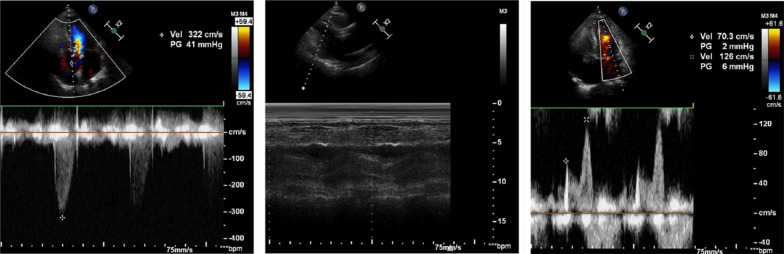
1 year follow-up after operation, echocardiography results
